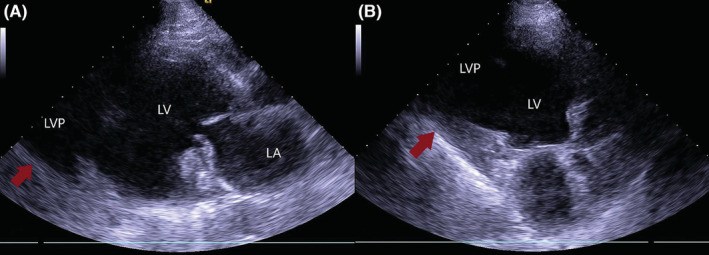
Transthoracic echocardiography showing an image of massive left ventricular pseudoaneurysm; (A) parasternal long axis view, (B) apical 4‐chamber view. LA, left atrium; LV, left ventricle; LVP, left ventricular pseudoaneurysm
